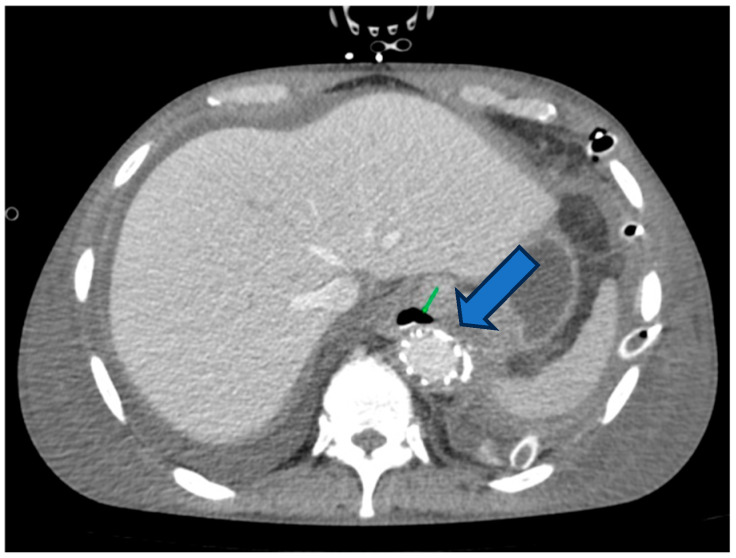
CT scan performed three weeks later, revealing the secondary esophageal fistula–endograft contact point.
